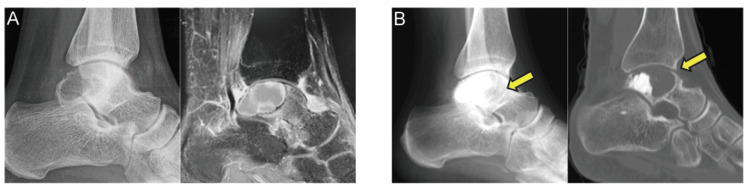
Case 1: initial images. (A) (Left) Radiograph shows an osteolytic change in the posterior talus. (Right) MRI demonstrates a talar bone tumor exhibiting rim enhancement on a gadolinium-enhanced T1 fat-suppressed image (right). (B) Findings of local recurrence six months after the initial surgery: (left) postoperative radiograph reveals osteolytic lesions surrounding the artificial bone graft (arrows) and newly developed osteoarthritic changes that were absent preoperatively. (Right) CT image shows talar dome collapse with osteoarthritic changes in the tibiotalar joint (arrows). MRI, magnetic resonance imaging; CT, computed tomography
